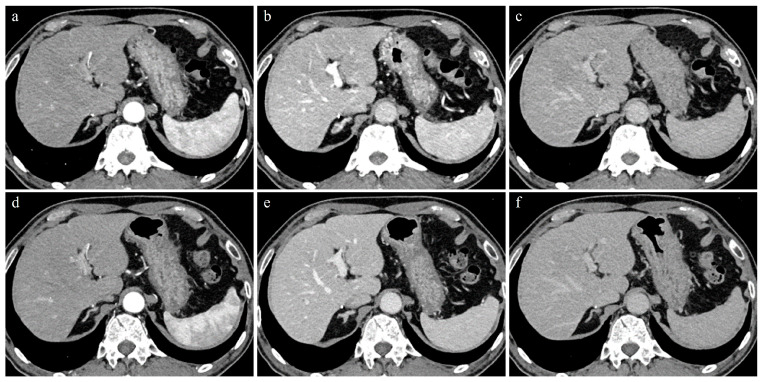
A 74-year-old man with 62.3kg body weight. (a–c) Initial CT images obtained with 70 kVp protocol during AP (a), PVP (b), and EP (c). (d–f) Follow-up CT images (9 months later) obtained with blended DE protocol during AP (d), PVP (e), and EP (f). All CT images were reconstructed by IR methods. Contrast enhancement of the hepatic parenchyma, portal vein, and hepatic vein in PVP and EP were better in 70 kVp than in blended DE protocol.
